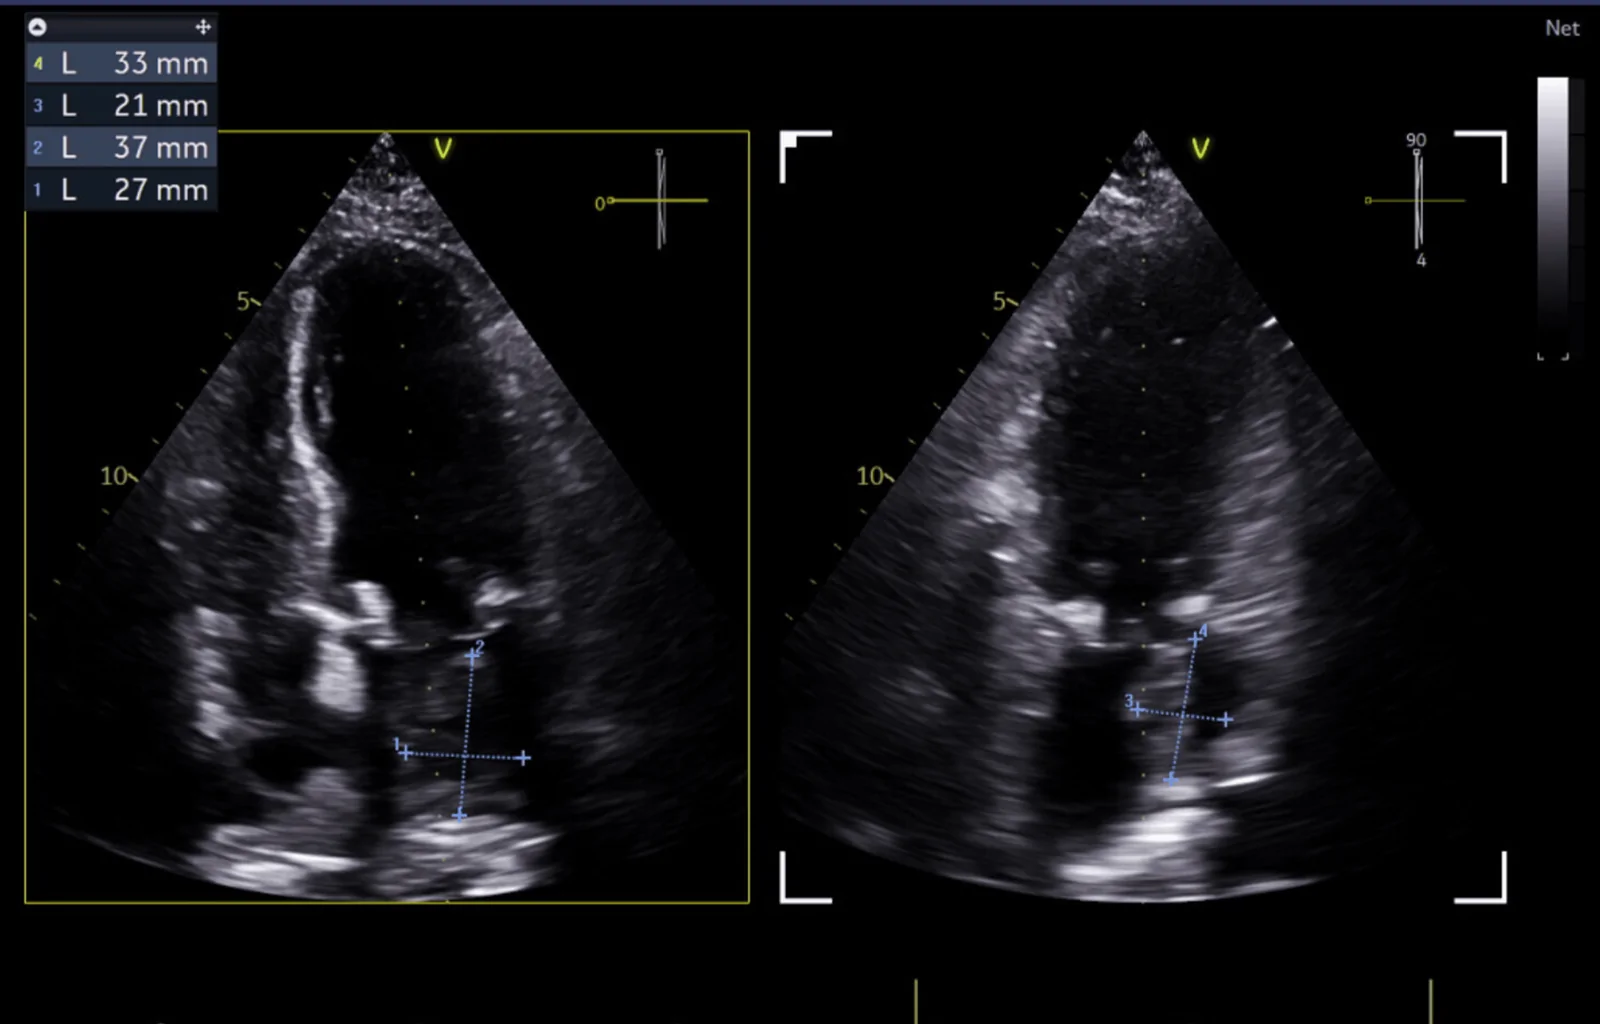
Postoperative transthoracic echocardiography (TTE) images of the patient Apical four-chamber (left-hand side image) and two-chamber (right-hand side image) views, demonstrating a left atrial hematoma along the posterior wall of the left atrium.
